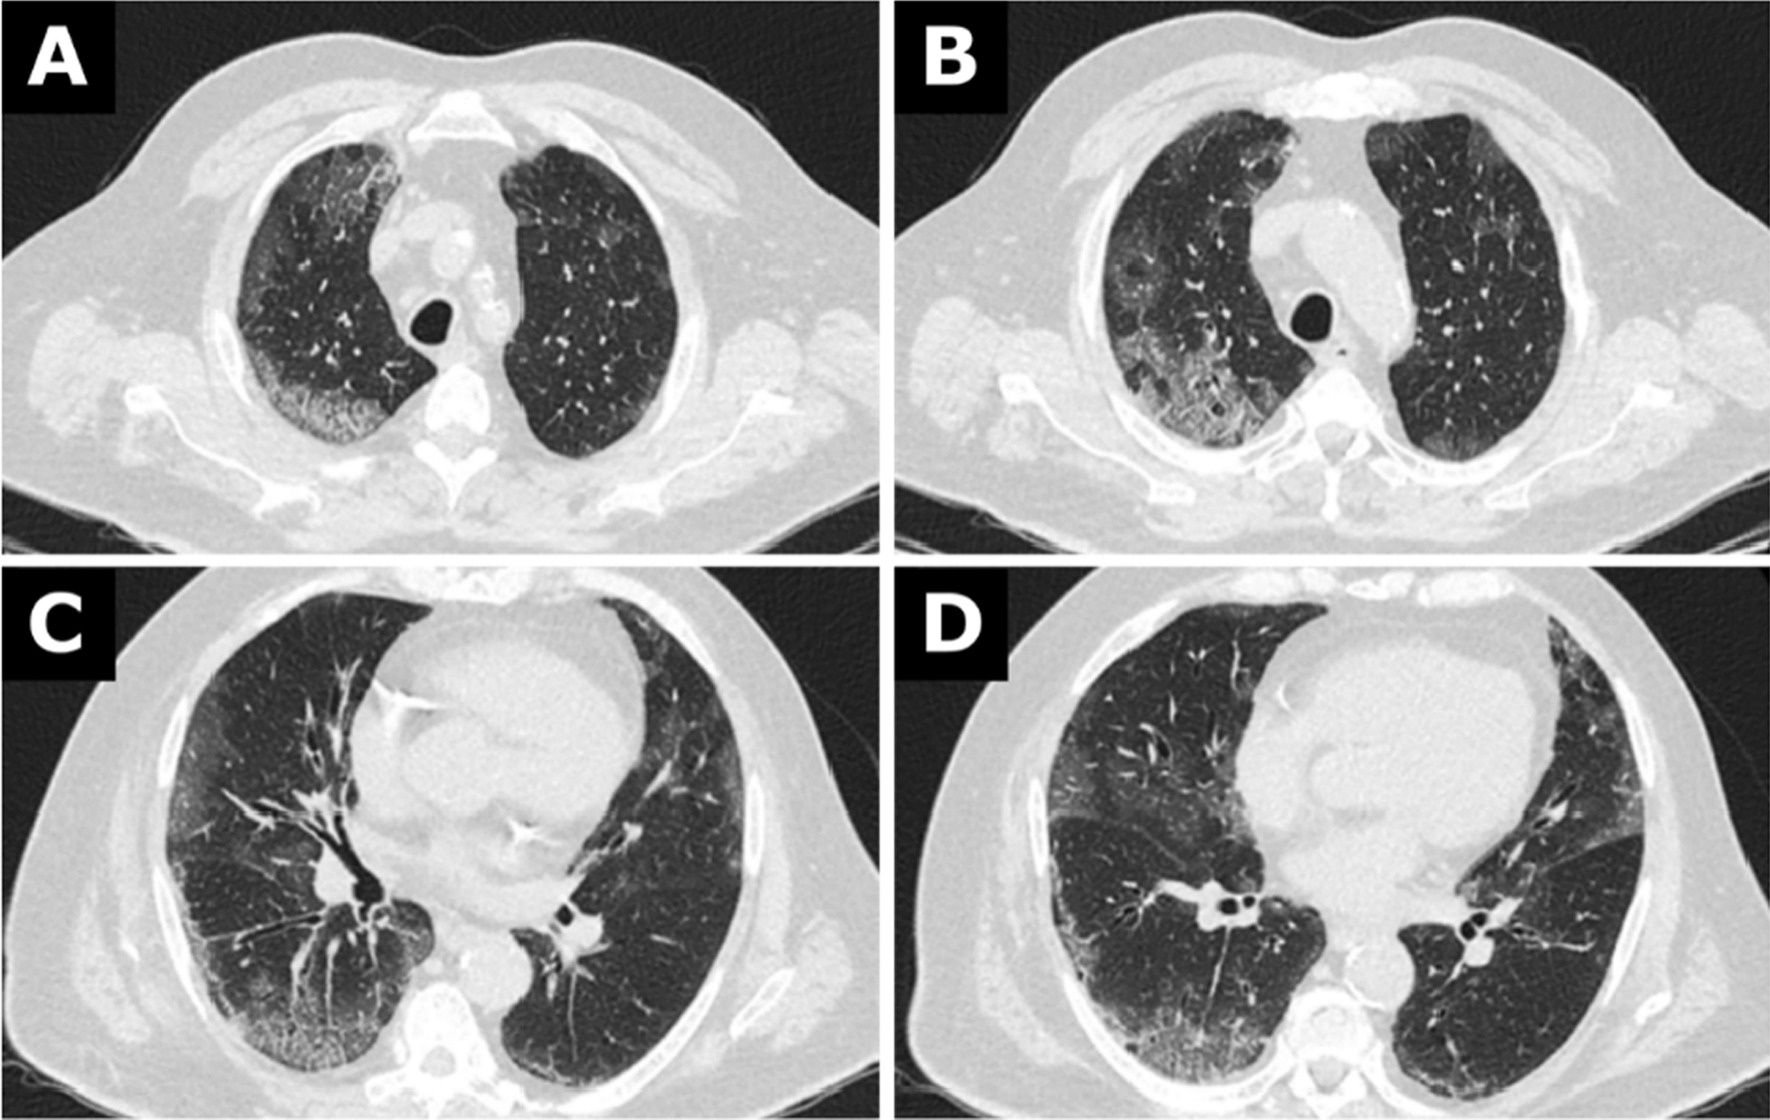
A–D Chest computed tomography from 30 December 2019
